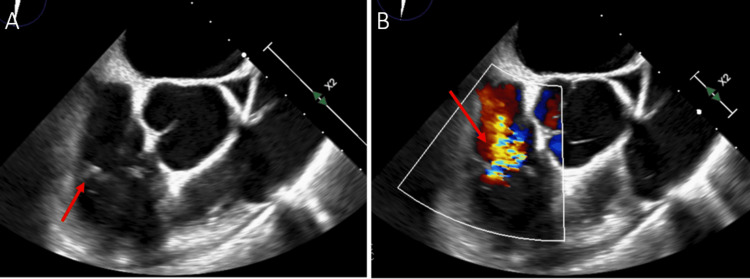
Transesophageal echocardiogram. (A) Demonstration of a thickened tricuspid valve with no evidence of endocarditis (by arrow). (B) Demonstration of moderate tricuspid regurgitation (by arrow).
